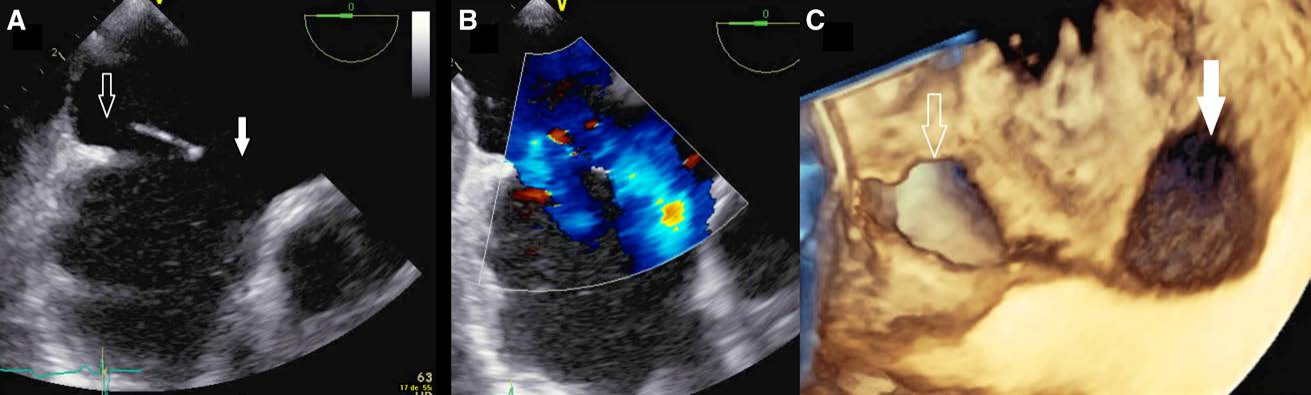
Transesophageal echocardiography showing 2 atrial septal defects. A, White arrow, secundum type atrial septal defect (ASD) in loco typico; white bordered arrow, ASD at the junction with the superior vena cava and the right upper pulmonary vein. B, ASDs with color Doppler showing left-to-right shunting. C, 3-dimensional reconstruction of both ASDs.
